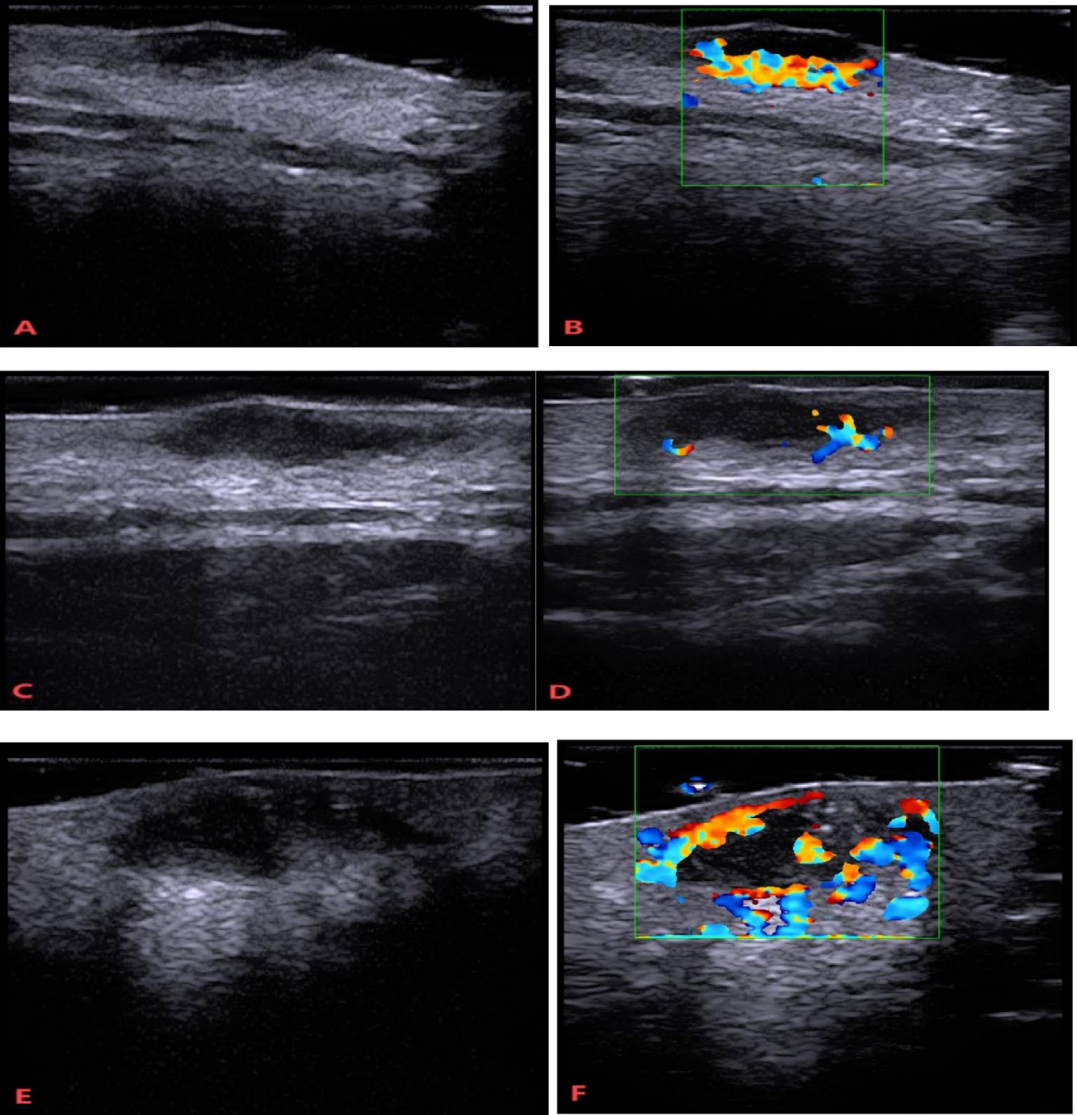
Representative sonographic images of fistulous lesions (A–F).
